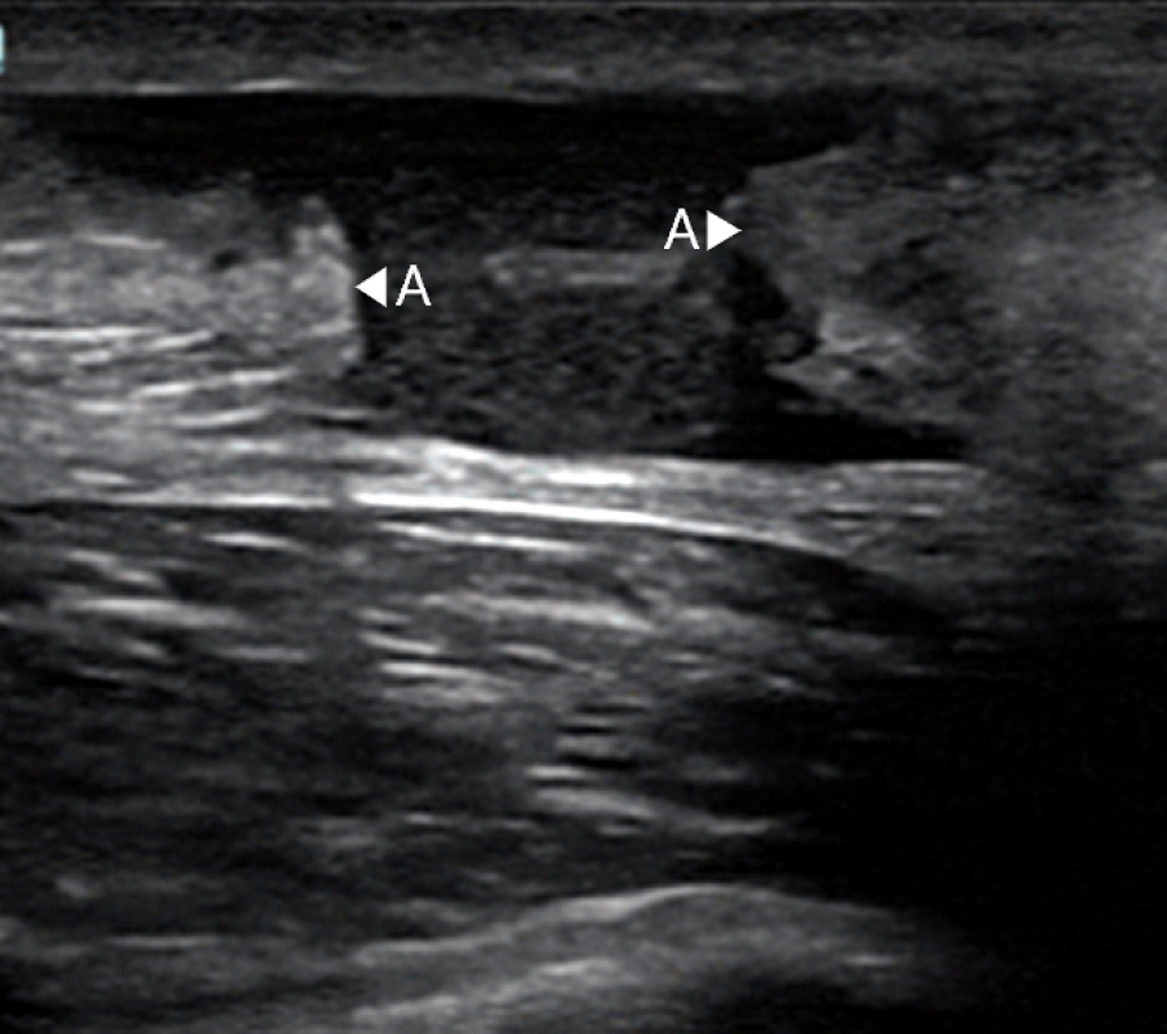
Demonstration of a full thickness Achilles tendon rupture in the longitudinal axis. Ultrasound image from Butterfield, 2020 [15] A- Disruption of Achilles tendon, visualisation of abrupt and thickened ends Original images authorised by respective owners
